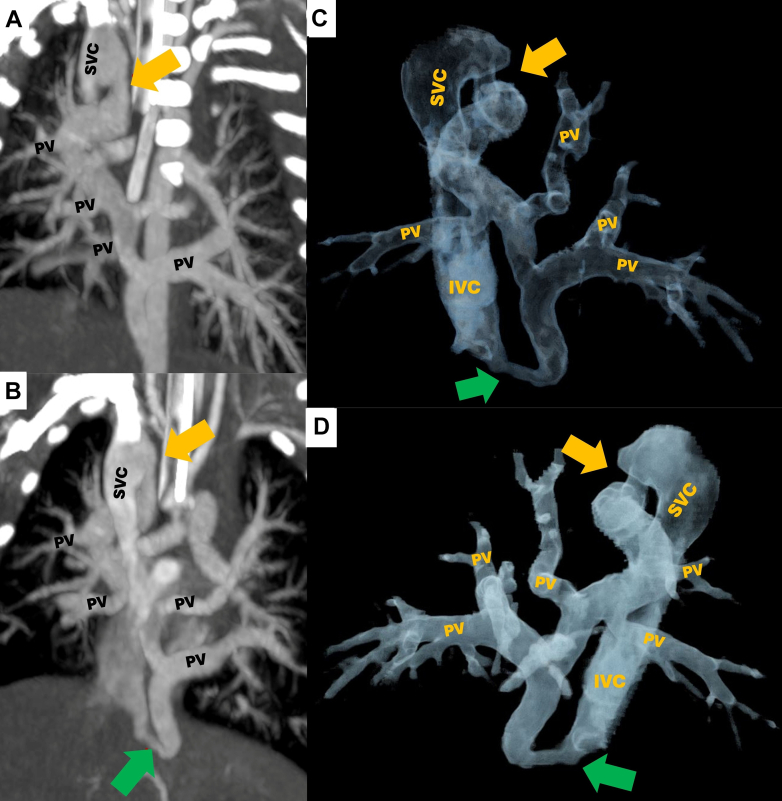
Patient 3 With Dual Drainage Total Anomalous Pulmonary Venous Connection Cardiac computed tomography angiography showing the right and left PVs draining independently into a retrocardiac collector with dual drainage: one branch to the SVC (yellow arrow) and the other (green arrow) to the confluence of the suprahepatic veins. (A, B) Coronal views using maximum intensity projection, (C) VRT 3D reconstruction in anterior view, (D) cinematic VRT 3D reconstruction imaging in posterior view. 3D = 3-dimensional; IVC = inferior vena cava; PV = pulmonary vein; SVC = superior vena cava; VRT = volume rendering technique.
